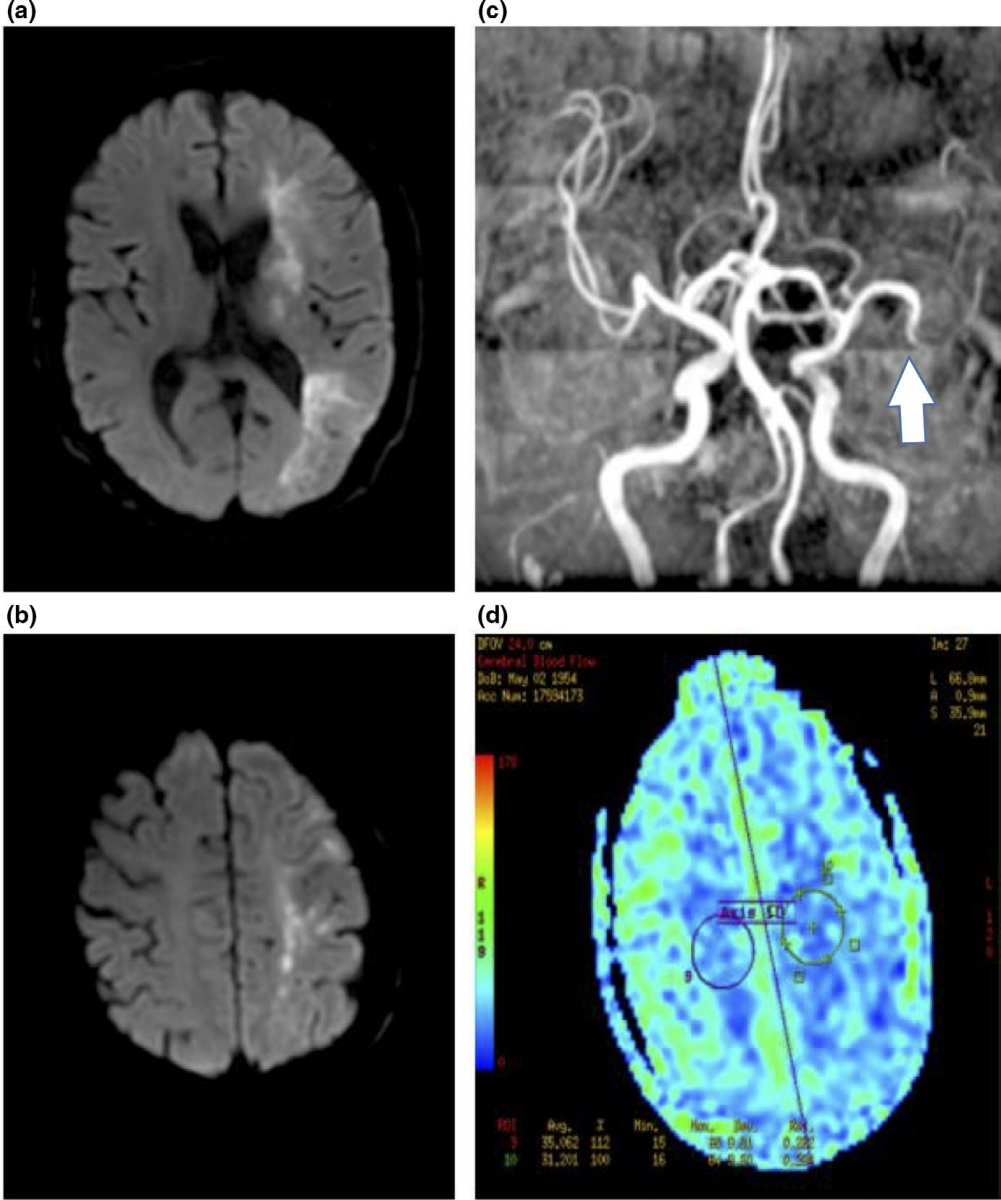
Mixed pattern stroke mechanism in ischemic stroke patients with ICAS. Images from a 64‐year‐old man with acute cerebral infarction in the left cerebral hemisphere. When the patient was admitted to the hospital, the consciousness was clear, presented with mixed aphasiathe, the right side of the nasolabial fold became shallow, right limb muscle strength was level 2, and the left limb muscle strength was level 5. NIHSS on admission was 18, and mRS at discharge was 4. (a,b) Multiple cortical infarctions in DWI indicate mixed mechanism of artery‐to‐artery embolism and hypoperfusion. (c) MRA shows occlusion of left middle cerebral artery. The arrows indicate stenosis location. (d) Arterial spin‐labeling shows hypoperfusion in left hemisphere
